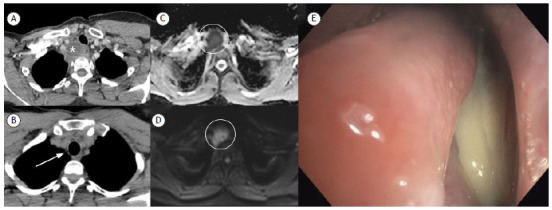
Panel A shows a hypodense mass (asterisk) in the right tracheoesophageal sulcus with mild peripheral enhancement and adjacent fat densification, suggestive of an abscess. A 9-year prior chest CT revealed an uncomplicated diverticulum at the same site (arrow in panel B). A diffusion-weighted MR image (panel C) and an apparent diffusion coefficient map (panel D) also confirmed the diagnosis of an abscess (circle in both panels). A bronchoscopy image (panel E) shows purulent secretion in the airway.
